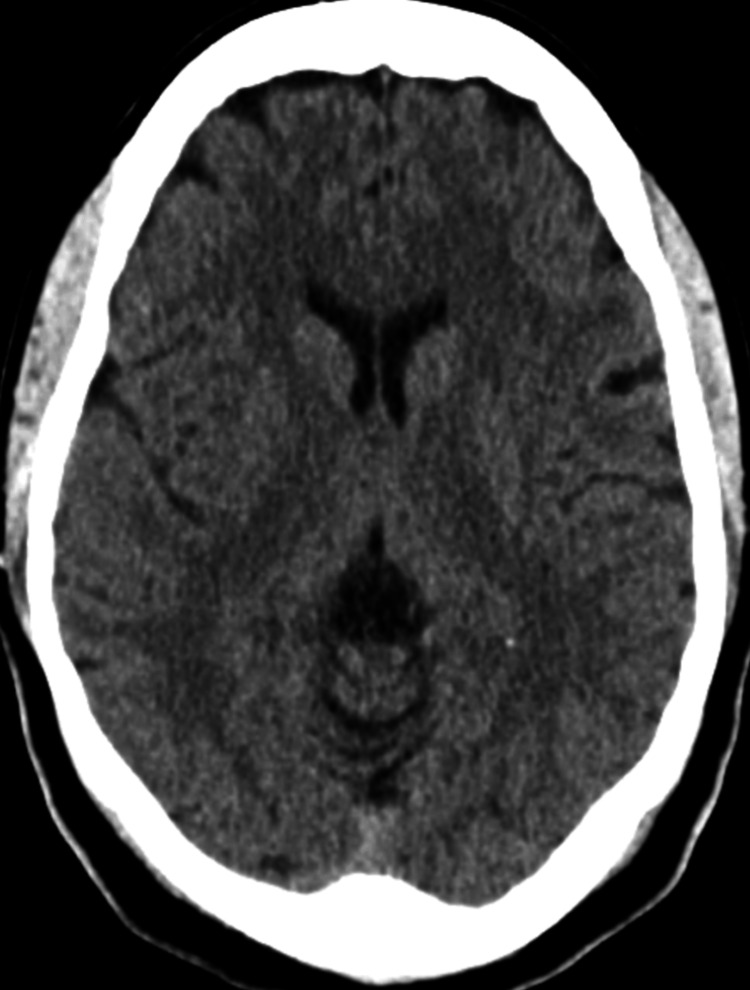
Cranial CT scan 2 Additional axial cranial CT image of the basal ganglia demonstrating no signs of intracranial hemorrhage.
